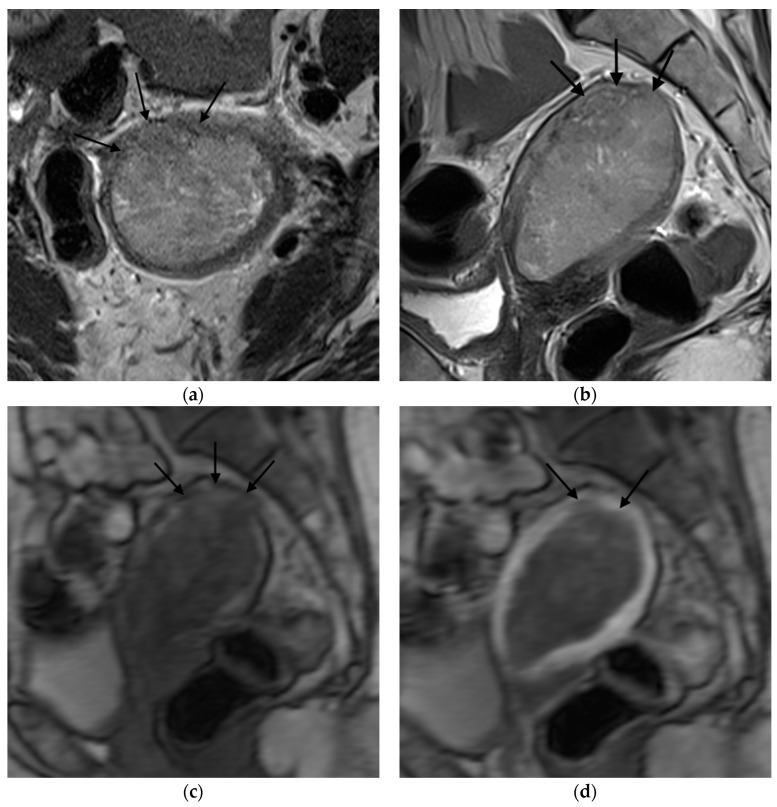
Representative MRI images of endometrial carcinoma with ≥50% myometrial invasion. (a) Axial T2WI, (b) sagittal T2WI, and (c,d) sagittal DCE-MRI images during the early (c) and late (d) acquisition stages. Tumors (arrow) with ≥50% myometrial invasion presented as iso- to mildly hyperintense on T2WI compared to the myometrium (a,b) with disruption of the junctional zone. On DCE-MRI, the tumors appeared as hypointense masses compared to the adjacent myometrium with interrupted subendometrial enhancement (SEE) (c), irregular tumor-to-myometrium interface, and extension of the tumor beyond the outer half of the myometrium (d). Abbreviations: MRI, magnetic resonance imaging; dynamic contrast-enhanced magnetic resonance imaging (DCE-MRI); T2WI, T2-weighted imaging; subendometrial enhancement (SEE).
